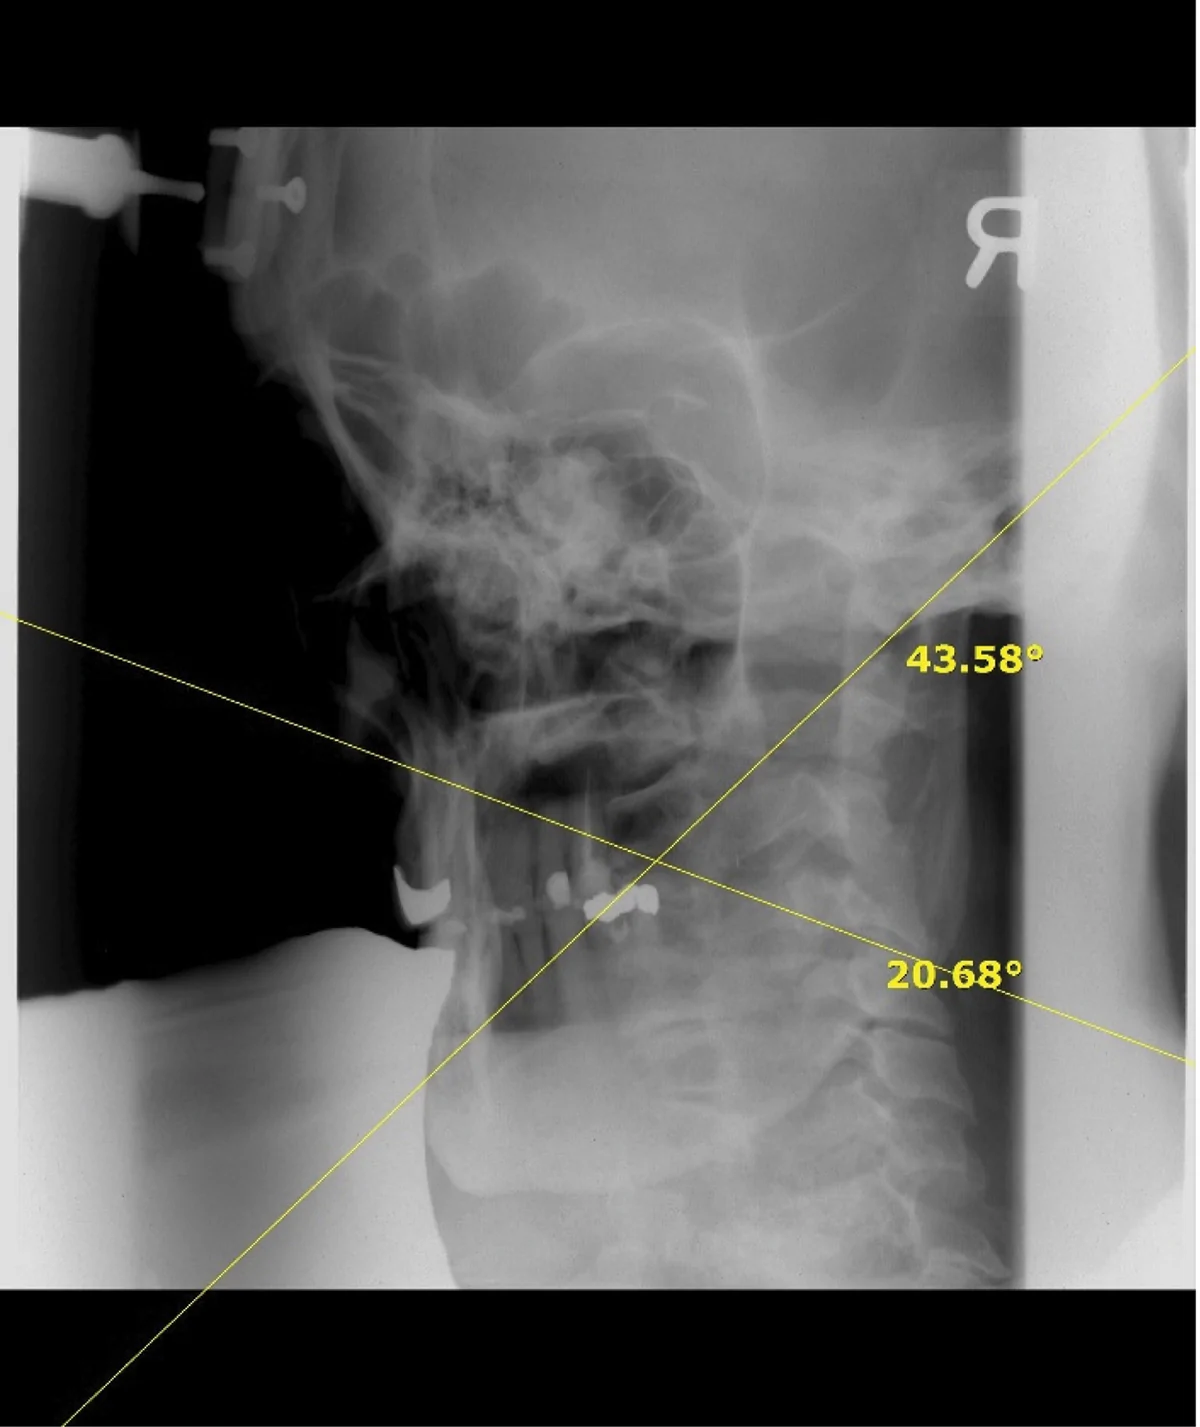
Left protracto view radiograph.
